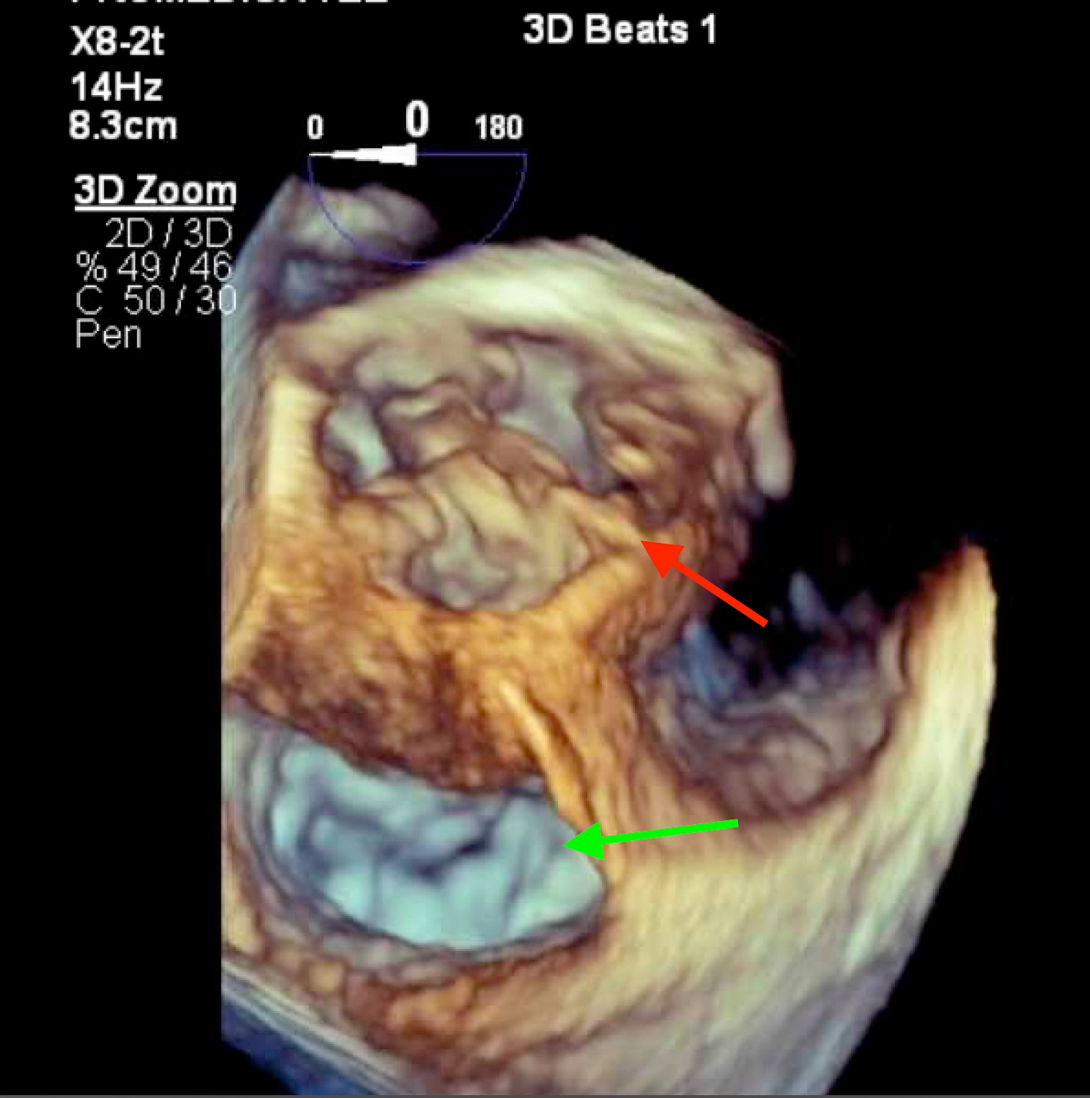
3D recreation on TEE showing vegetations on the aortic valve due to endocarditis and surrounding abscess formation (red arrow), mitral valve seen adjacent (green arrow). TEE, transesophageal echocardiogram
